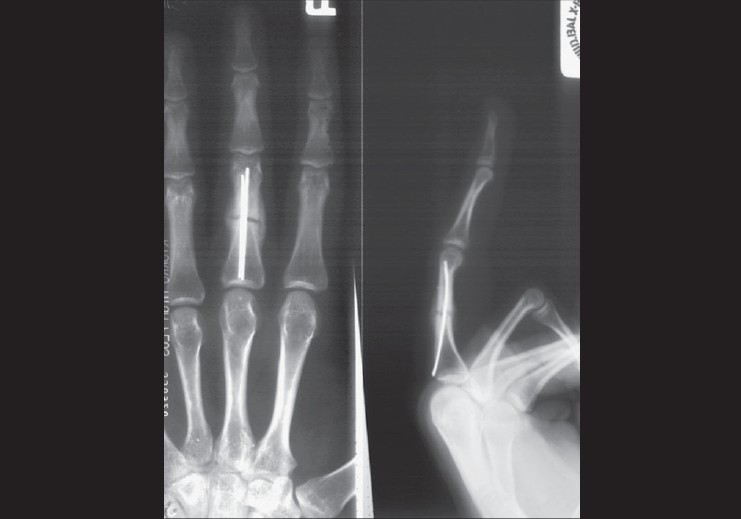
Anteroposterior and lateral X-ray views show radiological evidence of union in six weeks
